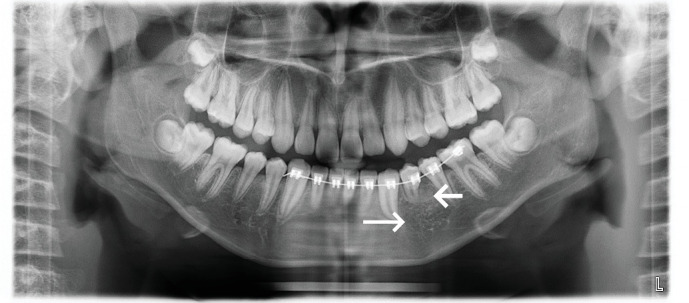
Panoramic radiograph was reexamined 6 months after exposure: panoramic radiograph showed that Tooth 34 had curved roots (short arrow), without obvious abnormality in the bone mineral density at the apical area (long arrow).
